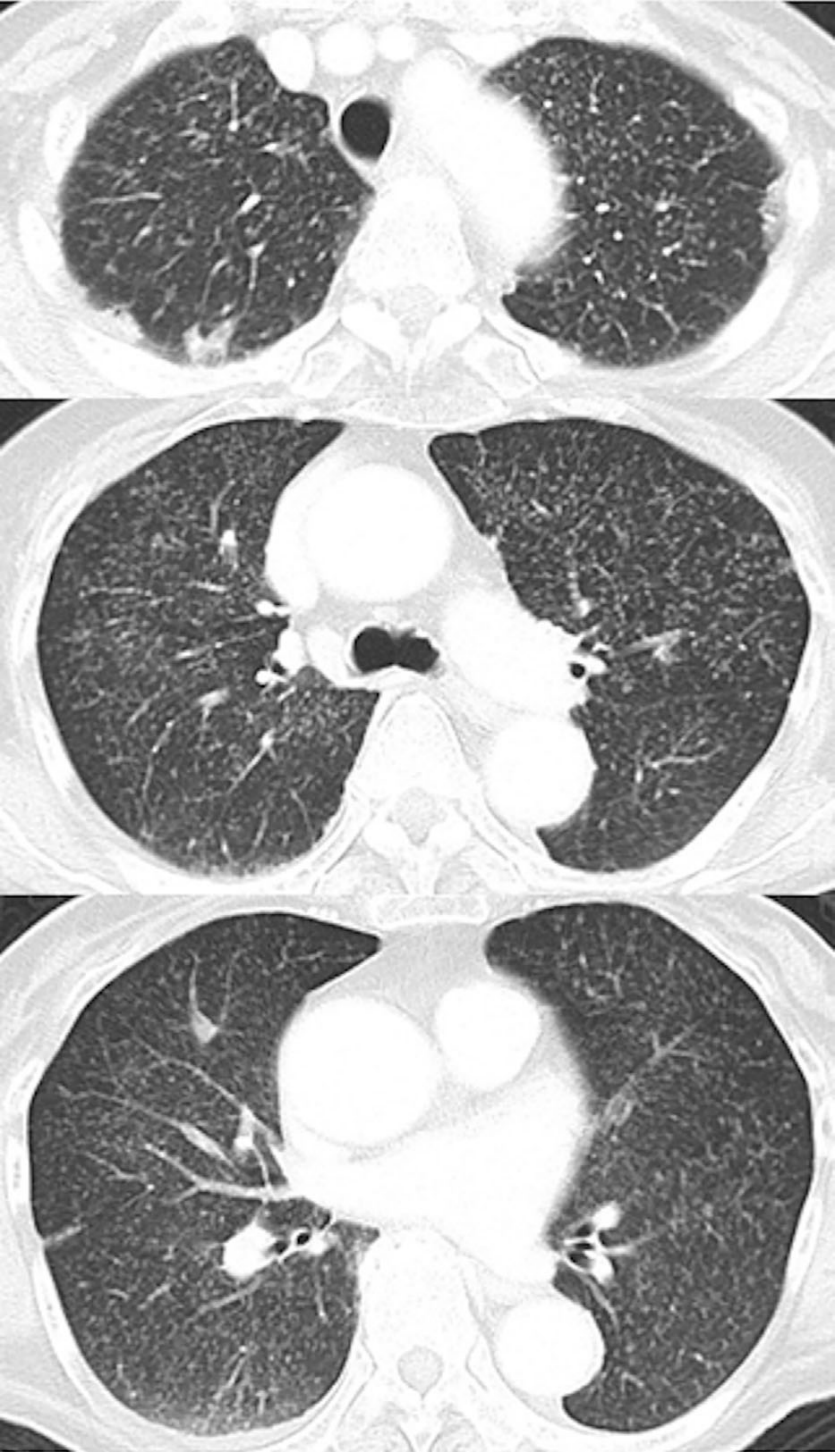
Chest computed tomography obtained at the third hospital 2 days before the present admission (day −2) showing diffuse granulation in both lung fields
